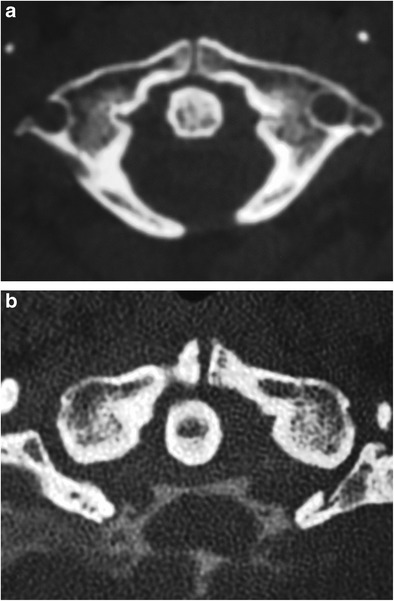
Hypoplasia/aplasia of the posterior arch of C1. Axial CT images at the level of the atlas on two patients reveal cervical vertebral cleft (posterior rachischisis) in *a* and absent posterior arches in *b*. Also note anterior vertebral body clefts on both images
